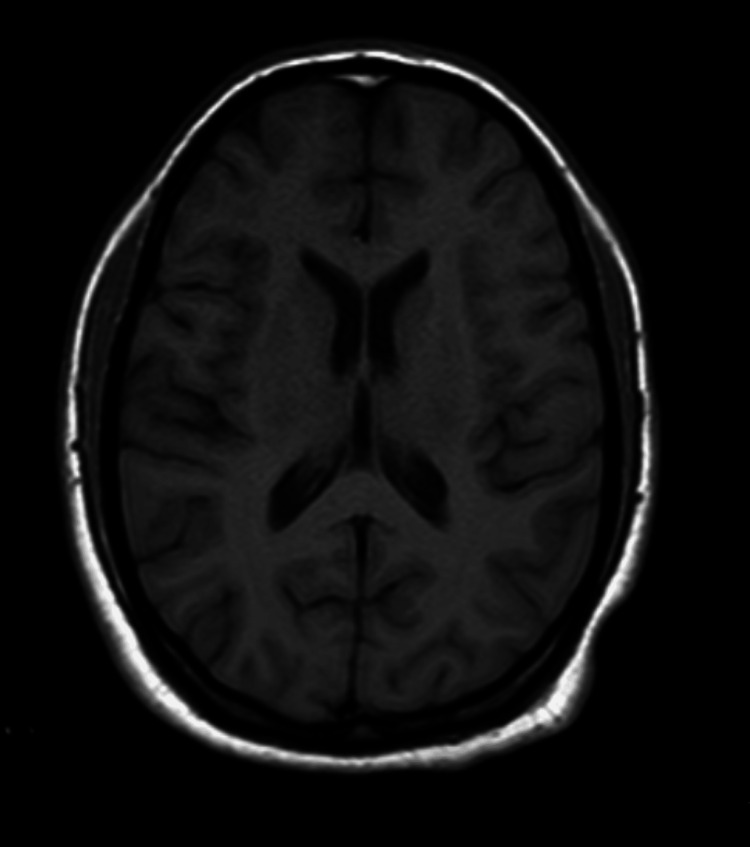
Normal contrast enhanced MRI brain No significant intracranial was abnormality noted.
